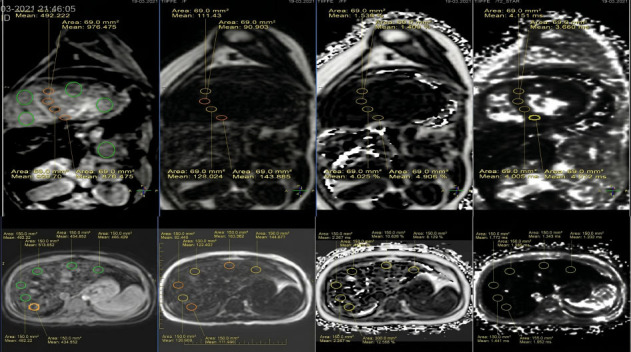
T2*MRI iron overload quantification, mean cardiac relaxation time was 4.7 and mean hepatic relaxation time was 1.4ms and mean cardiac iron concentration of 5.3mg per gram, and mean hepatic iron concentration of 17.8mg/g, suggestive of severe iron overload.
